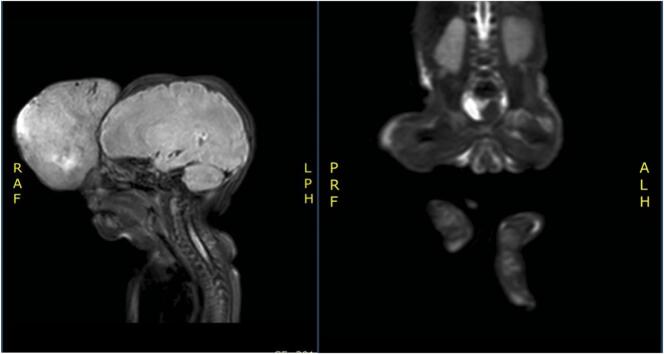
A. MRI of the forehead mass; B. proximal left thigh intramuscular lesion.
